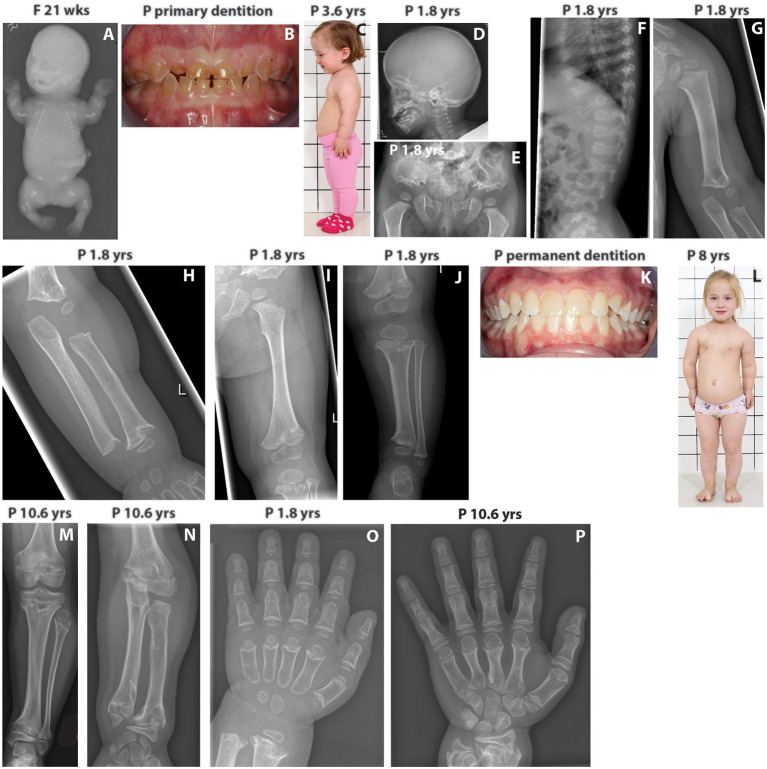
(A) Babygram of the deceased fetus shows extremely short tubular bones, very narrow thorax, horizontally oriented short ribs, and retarded ossification of the vertebrae. (B) Patient’s primary dentition showing dentinogenesis imperfecta. (C) The index patient at 3.6 yrs has short neck and limbs, redundant skin folds in the arms, and protuberant abdomen. (D) The skull of the index shows mild frontal bossing. (E) Patient’s pelvis showing trident configuration of the acetubulum and lacy iliac wings as well as short femoral necks with metaphyseal irregularities. (F) Patient’s spine displaying abnormal vertebrae with coronal clefts. (G–I) X-rays showing short and broad humerus with metaphyseal changes and shortening of the tibia and ulna as well as flared metaphyses. (J) The lower limb of the index patient displays short long bones, short femoral neck, marked metaphyseal irregularities, and normal epiphyses. (K) Patient’s permanent dentition with no signs of dentinogenesis imperfecta. (L) The index at the age of 8 yrs has normal facial features, short neck, redundant skin folds in the arms, narrow chest, prominent sternum, short limbs, mesomelic shortening of the upper arm and brachydactyly. (M) At 10.6 yrs, the index patient has marked metaphyseal irregularities of the tibia and ulna. (N) At 10.6 yrs, overgrowth of the fibula is noticed. (O,P) Hands of the index at 1.8 and 10.6 yrs. Brachydactyly and distinctive changes with deeper cupping of the metaphyses are evident. Progressive metaphyseal changes of the distal radius and ulna are also seen at 10.6 yrs. F, fetus; P, index patient; wks, weeks; yrs, years.
